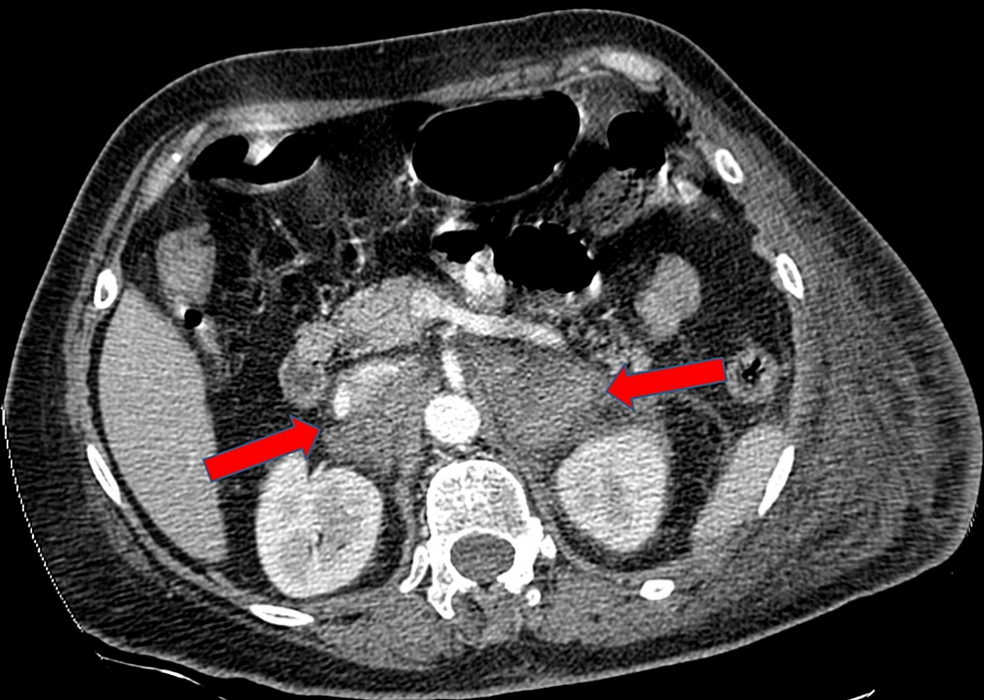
CT scan confirms bilateral adrenal hemorrhage. Adrenal congestion with adrenal thickening and periadrenal stranding. The adrenal hematoma appears oval with surrounding stranding of the periadrenal fat.
